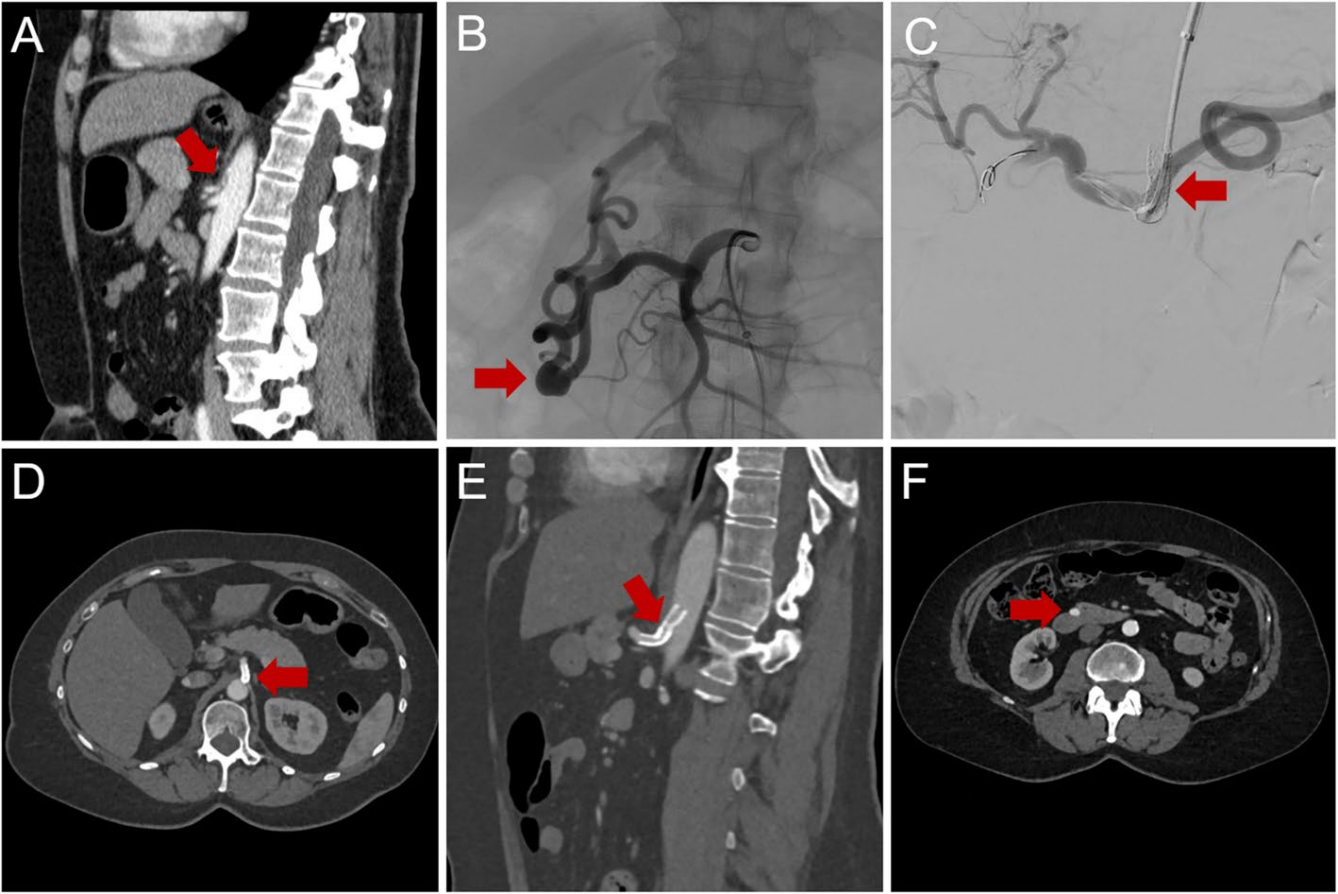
Representative case. CTA and DSA imaging of a 58-year-old female patient, who was referred to our clinic with upper abdominal pain. A Sagittal CTA reconstructions depict a subtotal occlusion of the CA (red arrow). B DSA showed a GDA aneurysm with an AVR of 2.6 (absolute size: 8 mm × 8 mm × 6 mm) in a critical position (red arrow). C Aneurysm rupture or active bleeding was not present. CA recanalization (red arrow) and a watch-and-wait strategy were performed. Follow-up CT was performed every 6 months in the first year and then every 12 months. D, E, F Follow-up CTA after 48 months shows patency of the CA stent (red arrows) and size constancy of the GDA aneurysm (absolute size: 8 mm × 8 mm × 6 mm) (red arrow)
